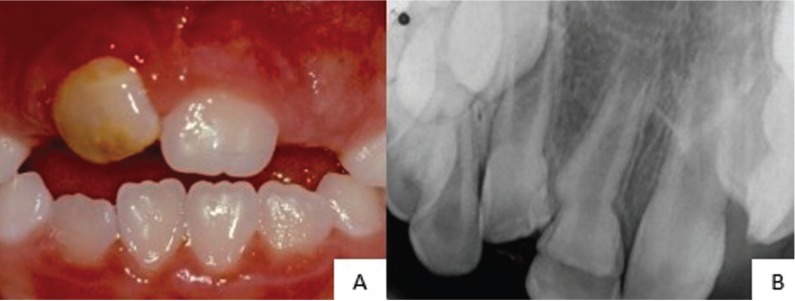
(a) Clinical presentation of the child at 8 years of age showing hypoplasia and crown dilaceration of the permanent upper right central incisor (b) Radiographic examination.
